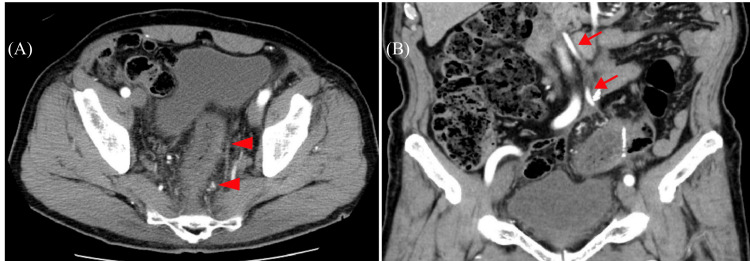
Contrast-enhanced computed tomography. (A) An edematous lesion and thickening (arrowhead) on the anal side of the anastomosis. (B) The inferior mesenteric artery (arrow) is not occluded.
